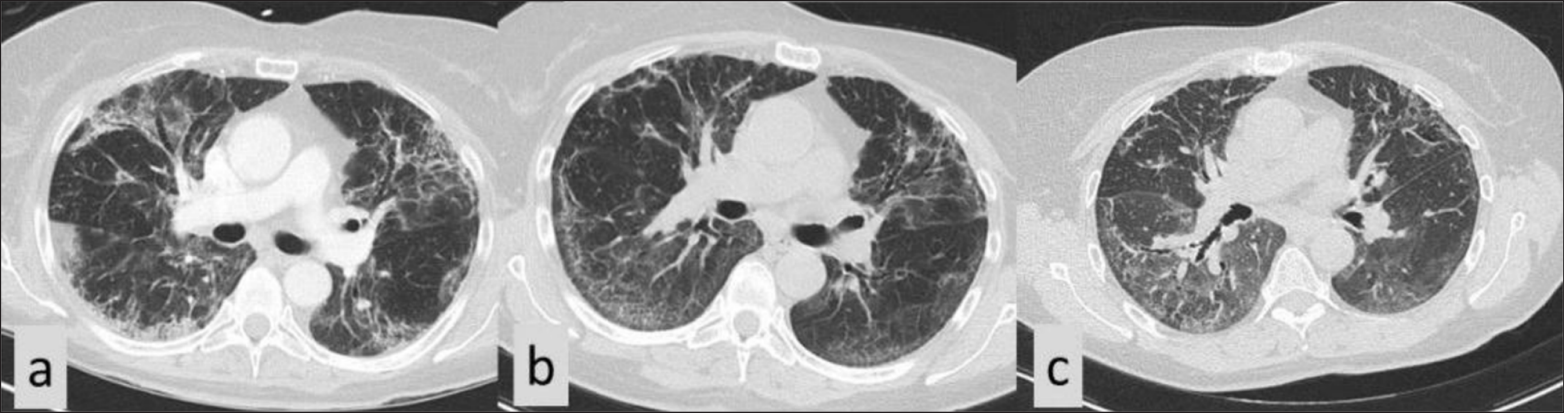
A 62-year-old woman showed on covid-scan multiple areas of peripheral ground-glass opacities in both lungs but also a more reticular pattern with interstitial thickening and irregular lines and bands (a). A control CT scan after 20 weeks showed the persistence of irregular lines and signs of interstitial fibrosis (b). A late control CT after 6 months showed the persistence of signs of interstitial fibrosis (c).
